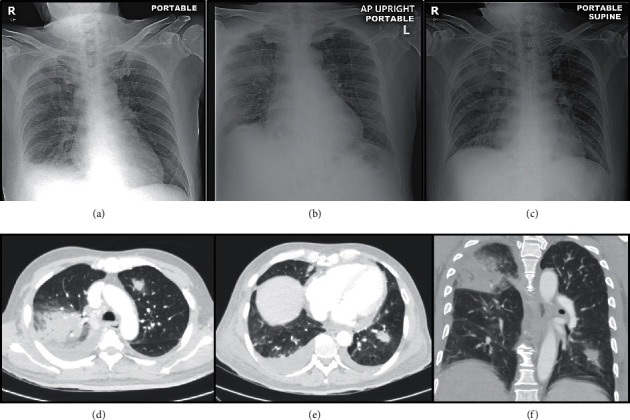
Images in a 58-year-old male patient with acute myeloid leukemia who had positive chest symptoms. (A) Baseline chest film shows right lower lung opacity. (B) Chest film on first day of febrile neutropenia (ANC = 0 *μ*L) shows new opacity at right upper lung and left retrocardiac region. (C) Chest film on the fifth day of febrile neutropenia (ANC = 624 *μ*L) shows stable opacities at right upper and left lower lungs. (D–F) Axial and coronal CT chest on third day of febrile neutropenia (ANC = 432 *μ*L) reveals pulmonary consolidation at right upper lobe and a nodular lesion at left lower lobe. Both lesions are surrounded by a halo of ground-glass attenuation, suggesting angioinvasive pulmonary aspergillosis. This case was evaluated as true-positive chest radiograph and CT chest.
